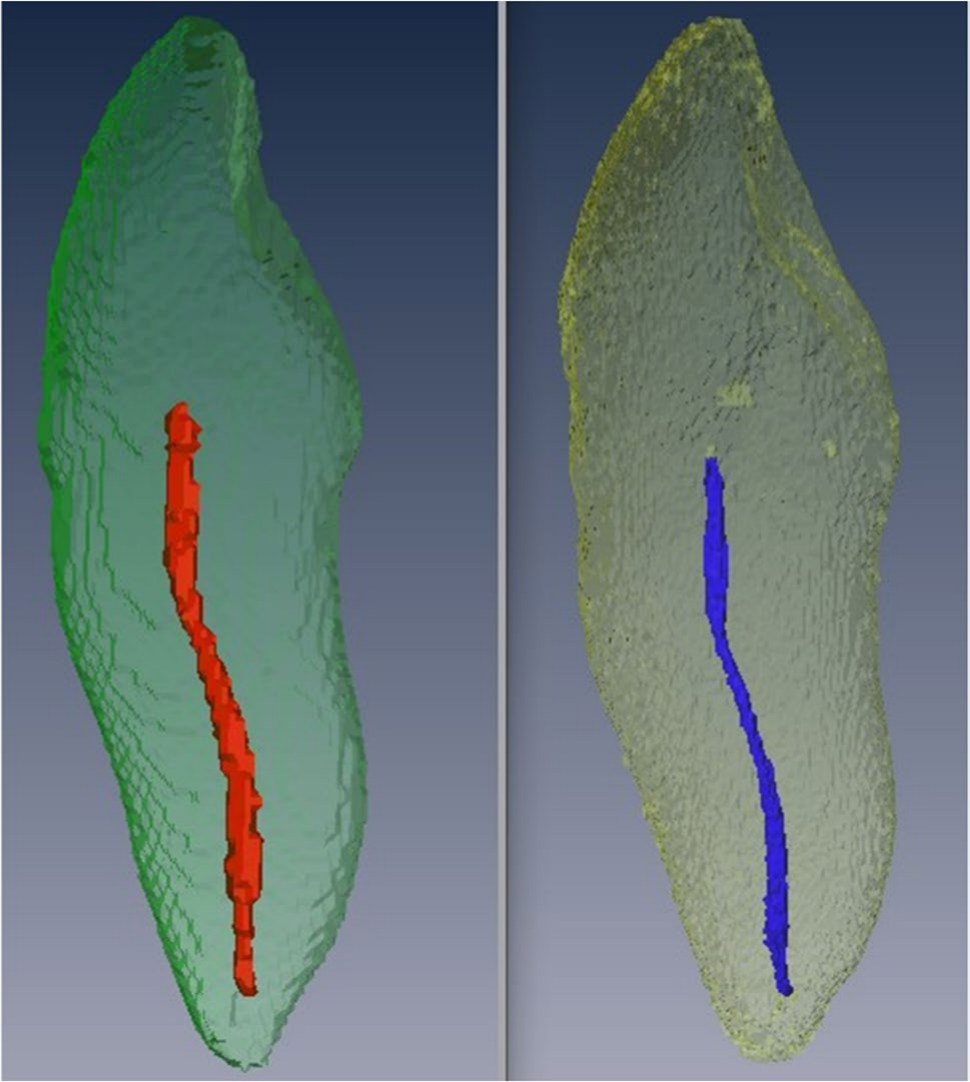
Tooth 11, 3D reconstruction, distal view. Left (red pulp): CBCT dataset, semi-automatic segmentation. Right (blue pulp): MRI dataset, semi-automatic segmentation. The impression of a larger pulp cavity reconstruction in CBCT is already visually striking
